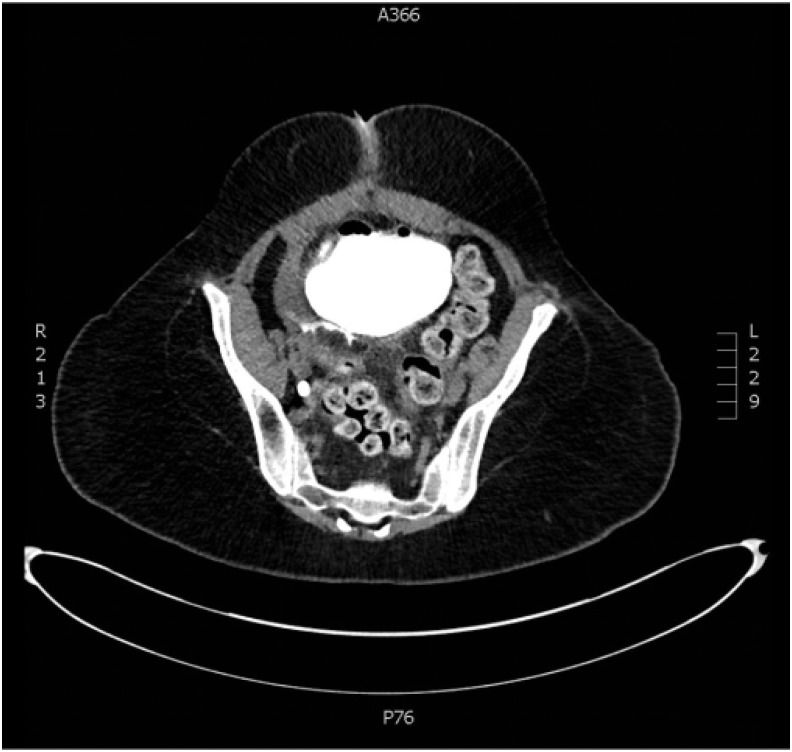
Axial imaging of CT cystogram showing posterior perforation with extravasation.
